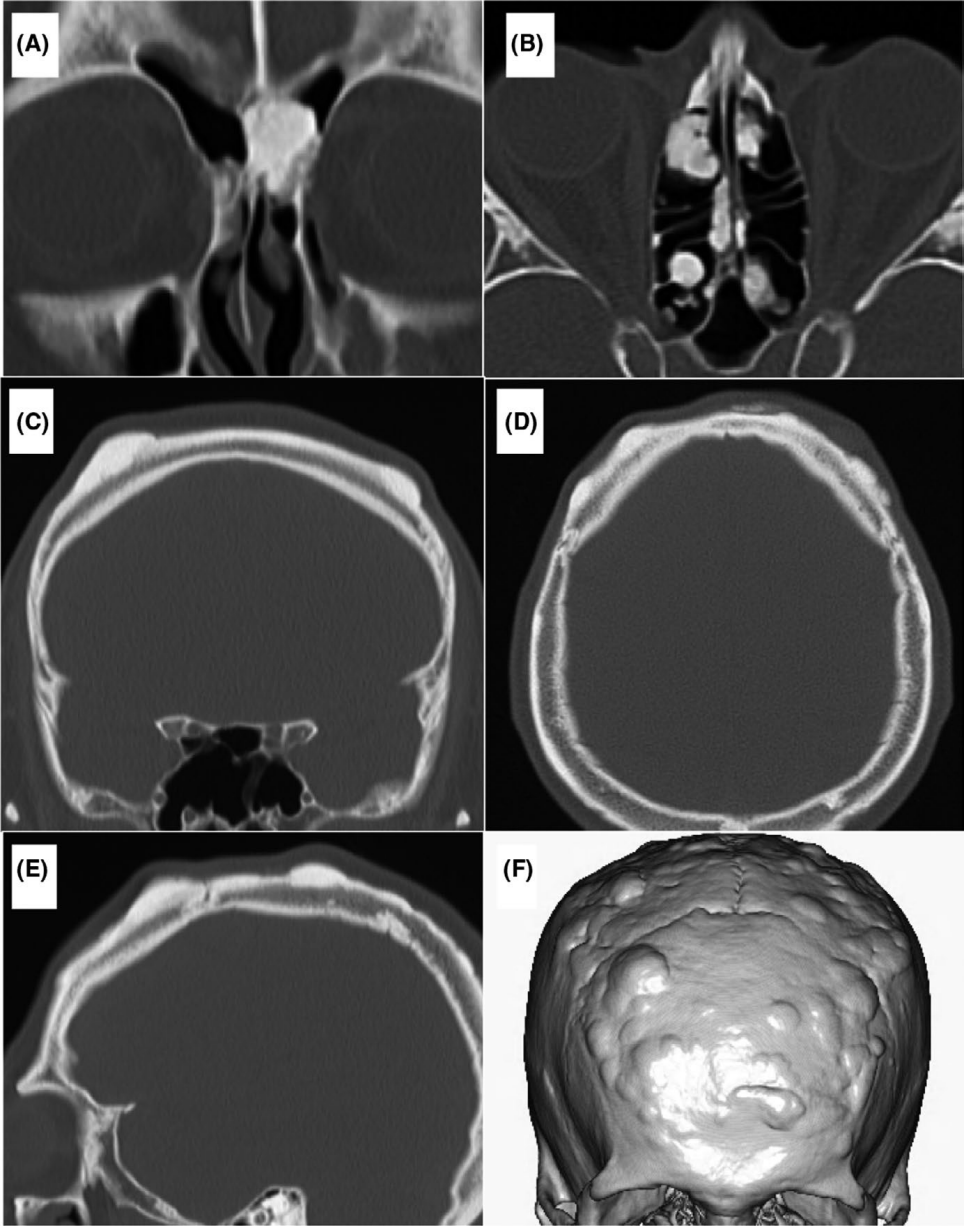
Initial computed tomography image shows multiple osteomas in the frontal sinus (A), ethmoid sinus (B), and skull (C, D, E, F)
